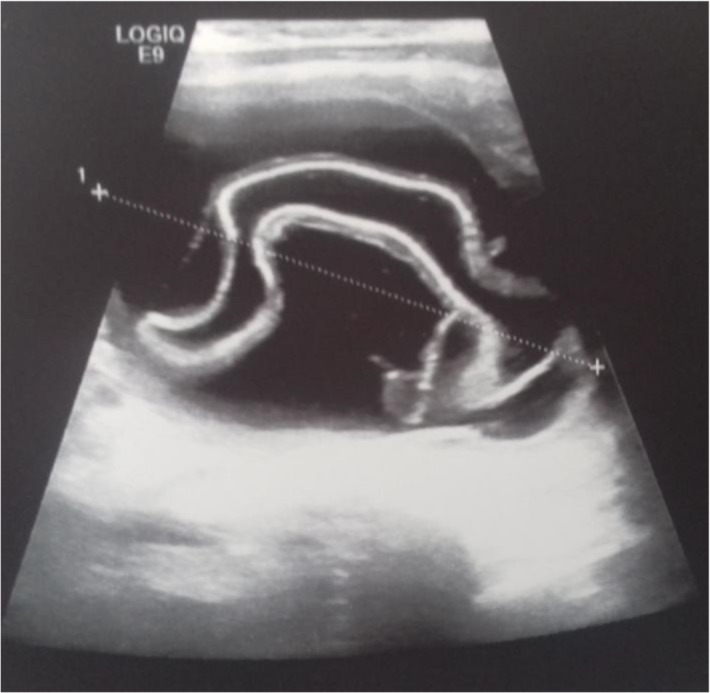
Abdominal ultrasound showed a well-defined, anechoic cystic mass, seat of a floating membrane
